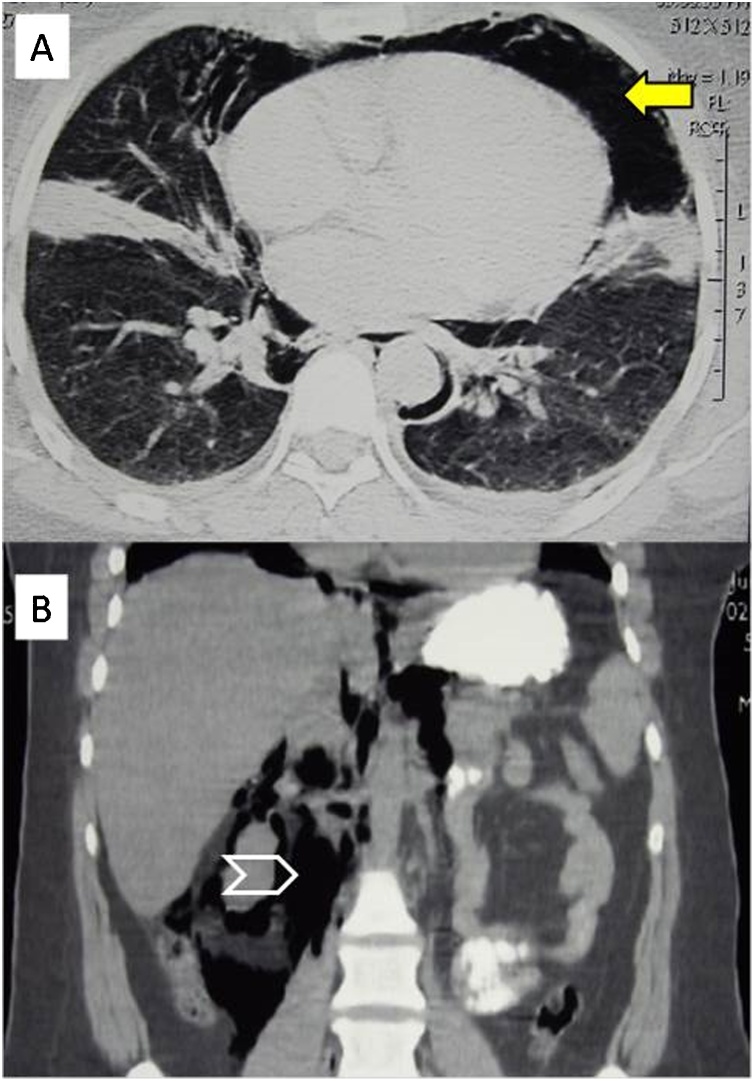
A 35-year-old woman presented with hypochondrial pain. Abdominal ultrasound showed thickening of the gall bladder wall and a dilated common bile duct of 8−10 mm. ERCP was tried but failed. The patient developed consistent upper abdominal pain following the ERCP. Chest CT scan (A) and coronal reconstruction of the abdominal CT scan (B) showed left pneumothorax (arrow) and retroperitoneal air (arrowhead).
